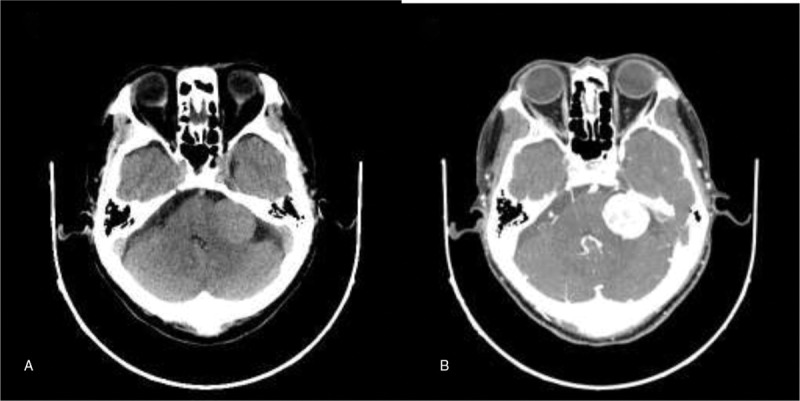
(A) Axial head CT revealed a 3.0 × 2.7 cm well-defined slightly hyperdense mass without peritumoral edema in the left CPA. (B) On enhanced CT, the mass was homogeneously enhancing, extending into IAC. CPA = cerebellopontine angle, CT = computed tomography, IAC = internal auditory canal.
